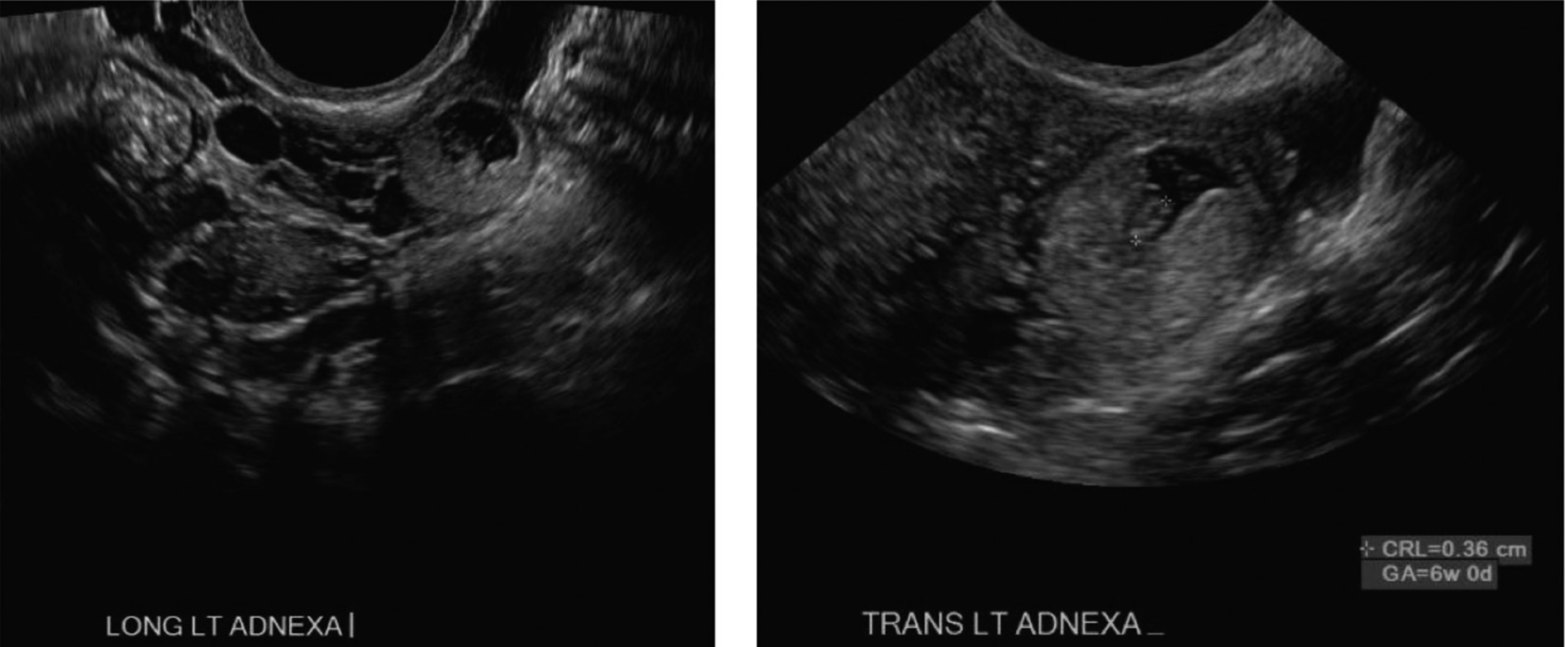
A. Transvaginal ultrasonography of left adnexal ectopic pregnancy demonstrating left ovary separate from ectopic pregnancy. B. Left adnexal ectopic pregnancy with crown rump length at 6 weeks 0 days.
